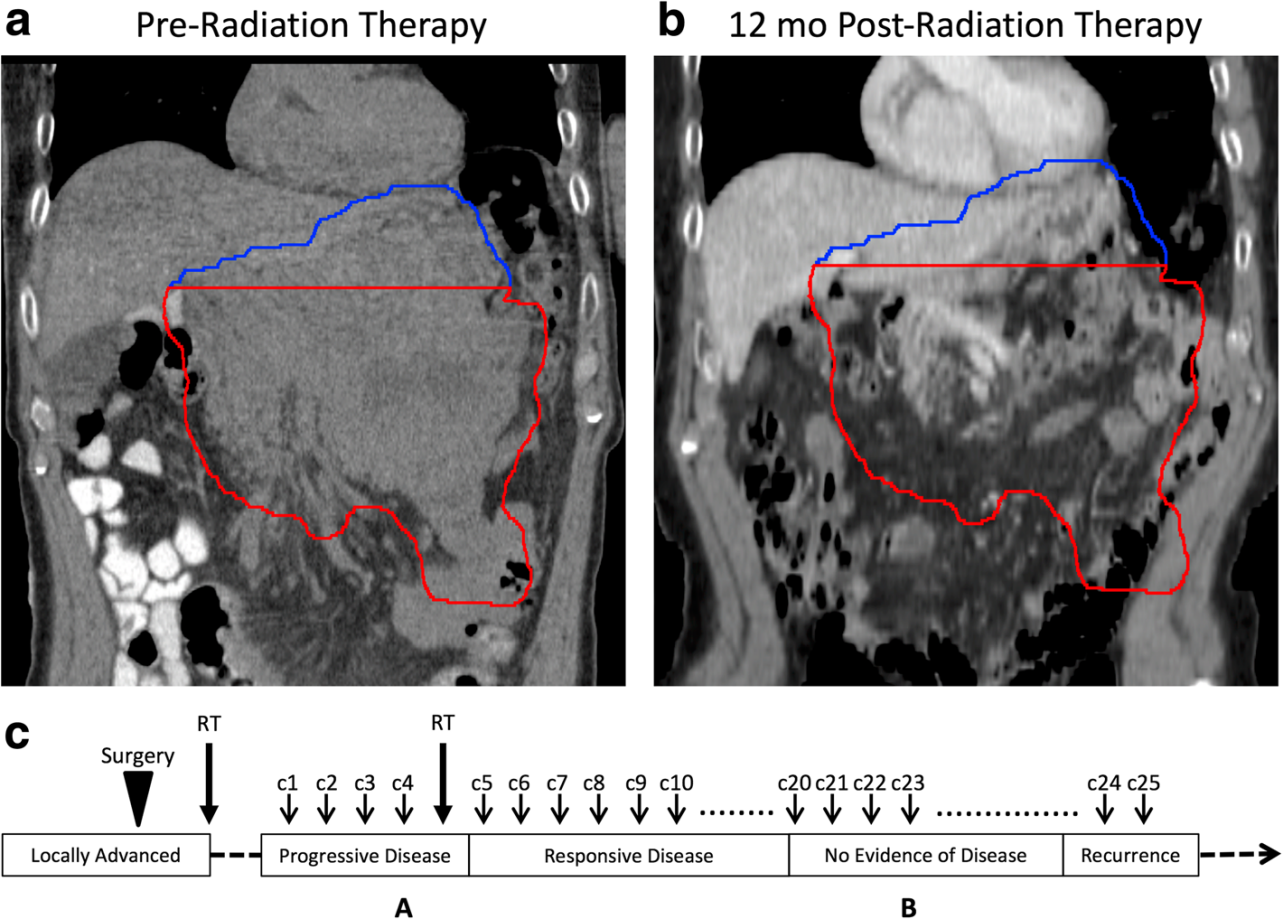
Case A. Abdominal metastasis before and after RT with target volume outlined in red and untreated disease outlined in blue. Left (Panel a): Coronal non-contrast CT scan obtained for RT planning demonstrating large abdominal mass. Right (Panel b): Coronal contrast CT obtained 12 months after RT demonstrating no evidence disease. Panel c: Timeline of therapy and disease status, with A and B corresponding to time points depicted in Panel a and b above. c1 = cycle 1 of pembrolizumab. CT = computed tomography. RT = radiation therapy
